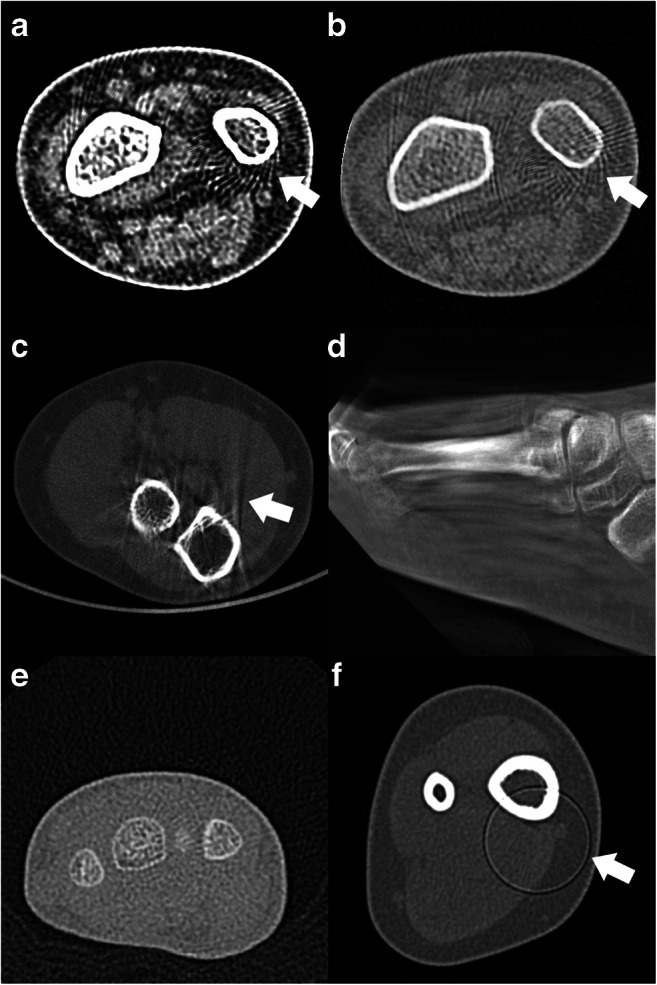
Examples of various CT artifacts. a and b Aliasing artifacts in an MDCT of the wrist. c Beam hardening artifacts in an elbow CBCT. d Motion artifacts in a CBCT of the foot. e Image noise in a wrist MDCT. f Ring artifact in an ankle MDCT
